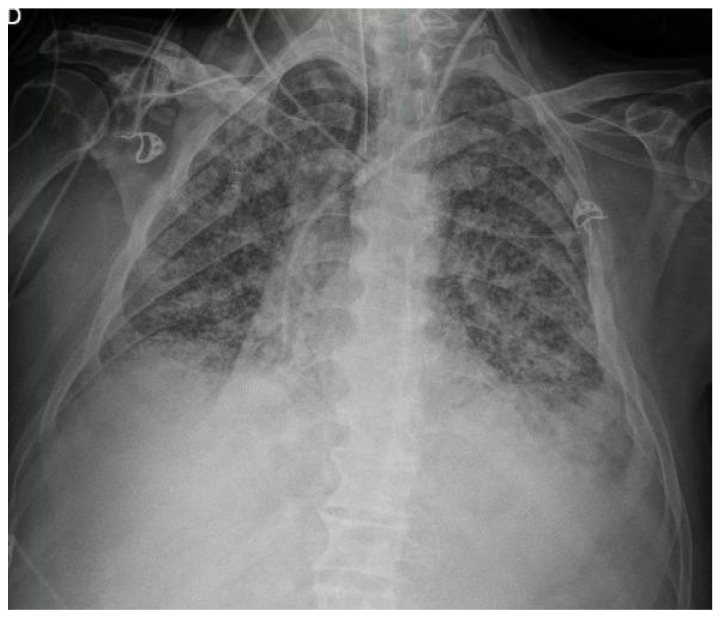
Chest radiograph obtained during the acute presentation, showing diffuse bilateral interstitial and alveolar infiltrates with a predominant perihilar distribution in an immunocompromised patient. Endovascular and airway devices are correctly positioned.
